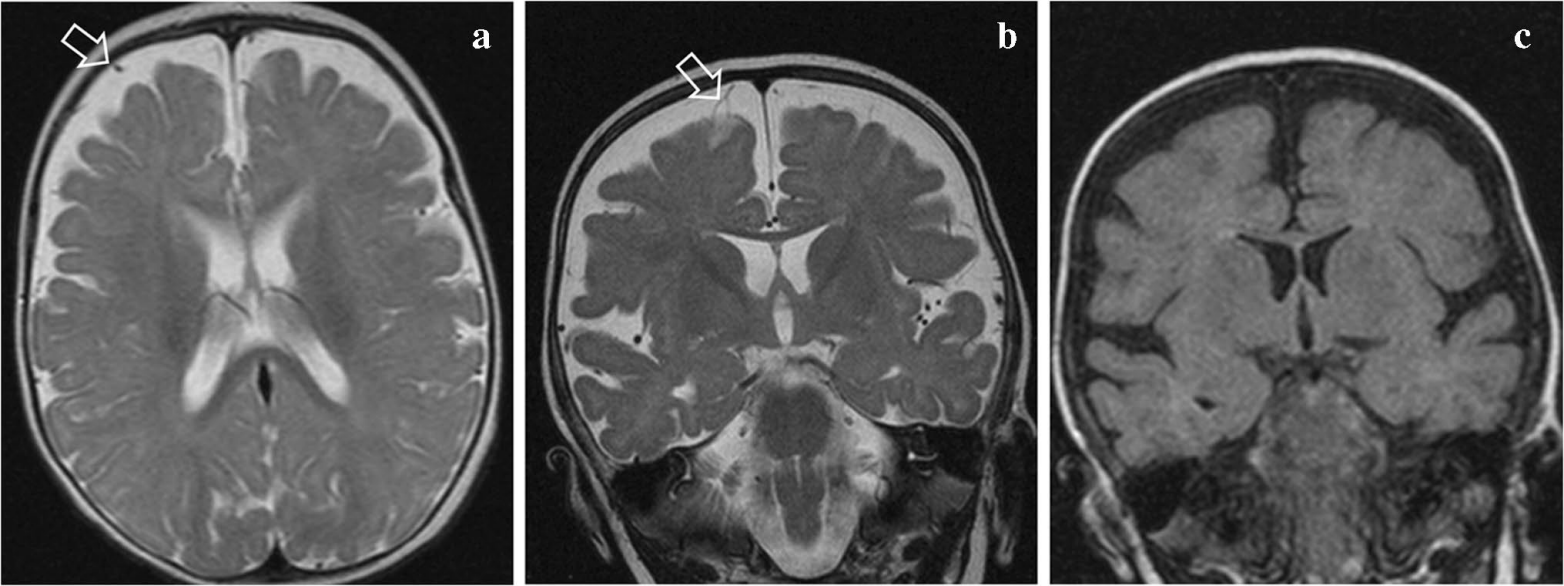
Brain magnetic resonance imaging of a 7-month-old male with a large head and seizures lasting less than 5 min. a Axial and (b) coronal T2-weighted scans show prominence of Cerebrospinal fluid (CSF) spaces over the frontal lobes with vessels traversing the entire width of the subarachnoid space (*arrows*). c Coronal fluid attenuation inversion recovery image shows extracerebral fluid with isointense signal compared to the CSF
